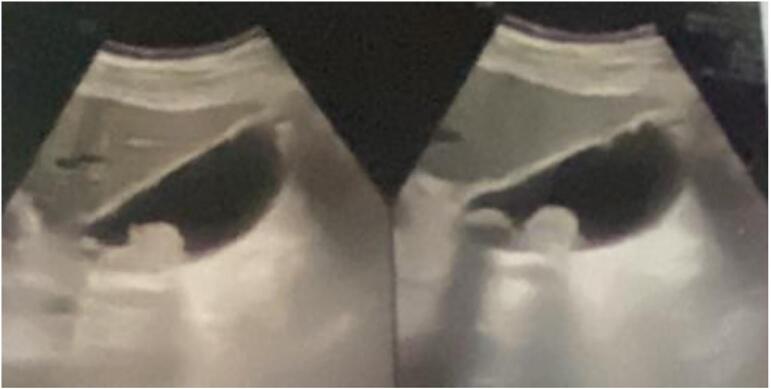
Ultrasound scan of liver and biliary tract. Inter hepatico diaphragmatic abscess. The gallbladder enlarged in size with thickened and edematous wall.
